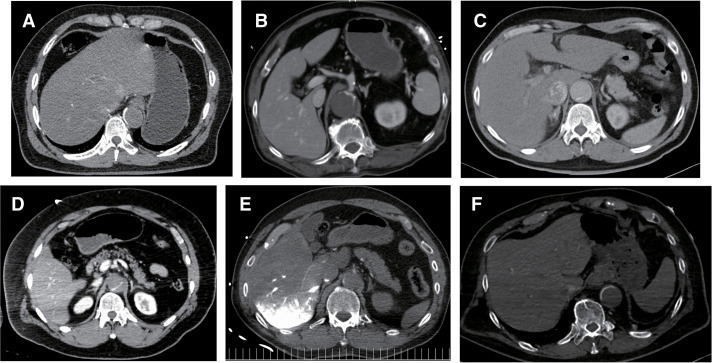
(A–F) Preoperative computed tomography images of each patient showing a collapsed true lumen.
